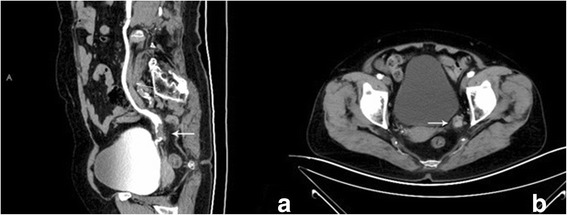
a Computed tomography urography shows an irregular mass in the left lower ureter. b The mass had a significant contrast enhancement during the nephrographic-phase
